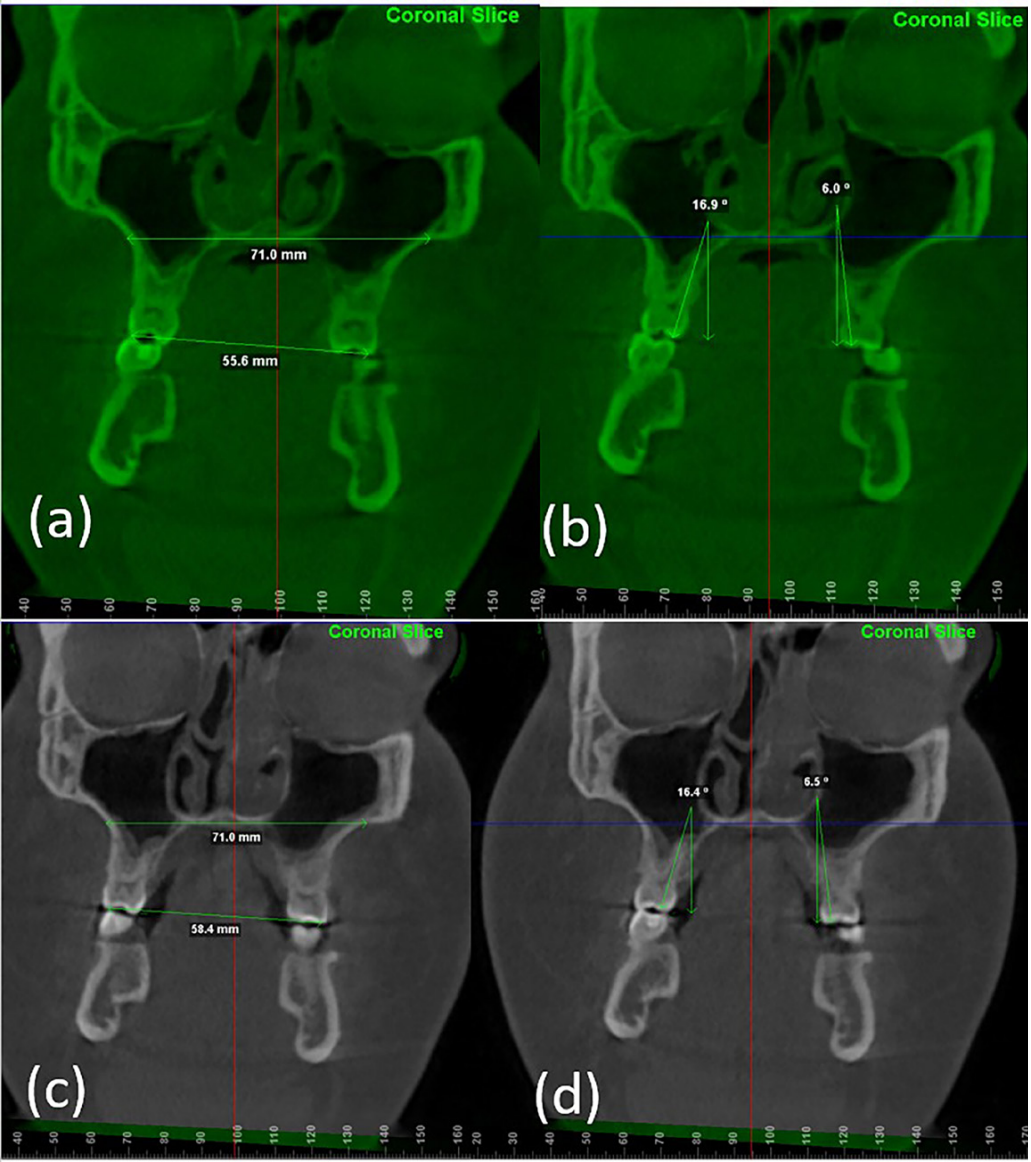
(a) Cone Beam Computed Tomography (CBCT) Face 2019: Coronal view. Inter-maxillary width. The intermaxillary width was maintained at 71.0mm. This measurement was taken from the outer cortex of the maxilla from left to right tangent to the hard palate, (b) CBCT Face 2019: Coronal view. The molar inclination measuring a line from palatal cusp going through the palatal root with a line perpendicular to the hard palate, (c) CBCT Face 2023: Coronal view. Inter-maxillary width maintained at 71.0mm. This measurement was taken from the outer cortex of the maxilla from left to right tangent to the hard palate superimposed with (a) to ensure measurement was taken in same plane of space. Improvement in the crossbite was achieved with the intermolar width changing from 55.6 mm to 58.4 mm, (d) CBCT Face 2023: Coronal view. The molar inclination measuring a line from palatal cusp going through the palatal root with a line perpendicular to the hard palate superimposed with (b) to ensure measurement was taken in same plane of space. The left maxillary first molar tipped buccally from 6.0° to 6.5° as expected. The right maxillary first molar decreased in angulation from 16.9° to 16.4°. Since there was improvement in the crossbite with the intermolar width changing from 55.6mm to 58.4 mm, the decrease in angulation post treatment was probably due to the torque built into the bracket system.
